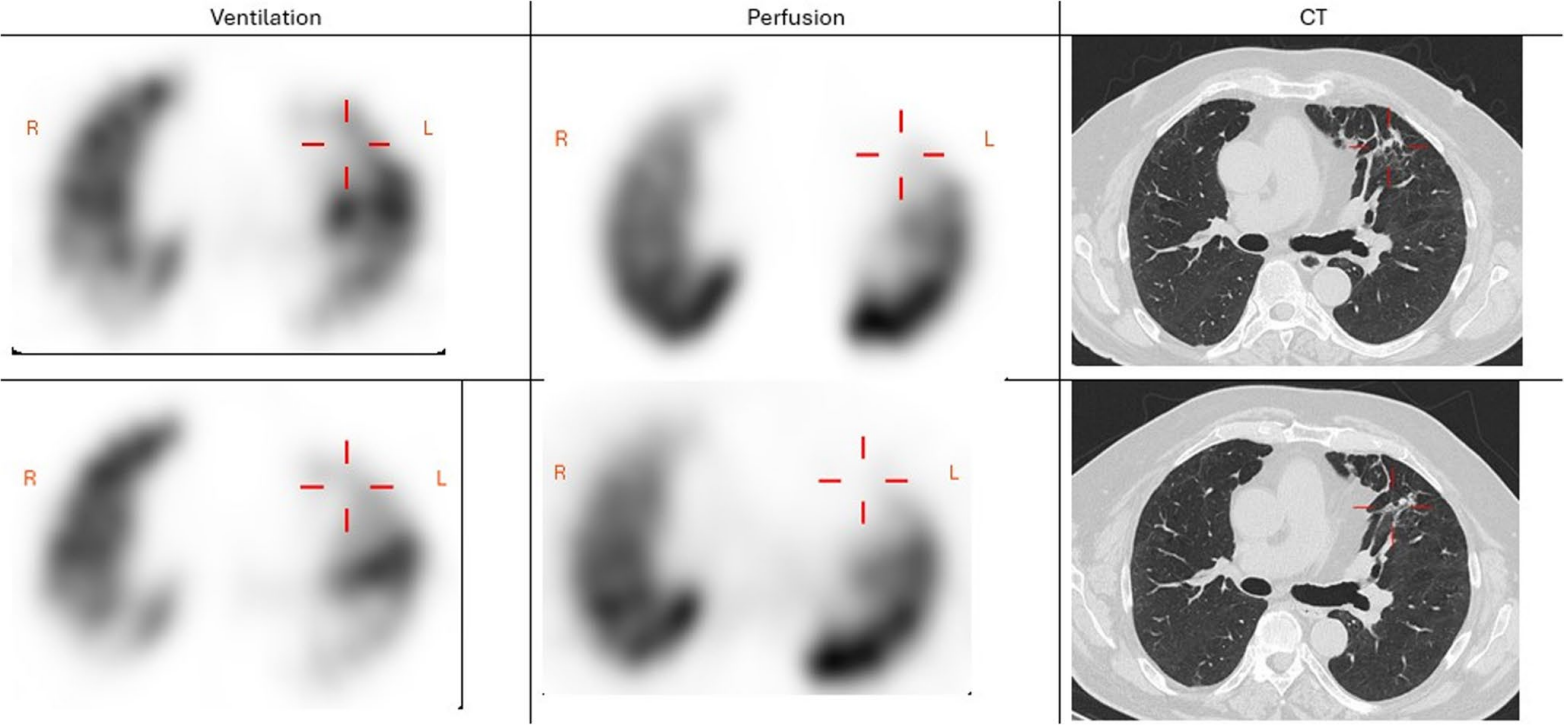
Chest imaging findings at short-term and intermediate follow-up in a 71-year-old male. Ventilation-perfusion single-photon emission computed tomography (V/Q SPECT) with high-resolution computed tomography (HRCT) shows a subsegmental mismatched perfusion defect with slightly reduced ventilation in the left segment 3 (red crosses on axial projections), both at short-term follow-up (6 months after COVID-19; upper row) and at intermediate follow-up (13 months after COVID-19; lower row). At both timepoints, HRCT shows reticulation, fibrosis and emphysema, but these are less pronounced at intermediate follow-up, indicating that the mismatched perfusion defect is caused by fibrosis rather than thromboembolic disease. Also, hypoventilation with inverse mismatched ventilation defects are seen in the posterior parts of both lungs at both short-term and intermediate follow-up. Overall, the chest imaging findings are compatible with a primary ventilatory disturbances due to fibrosis-like changes in the lung. Accordingly, lung function testing showed moderate restriction with a total lung capacity that was 67% of predicted at both time points, while the forced vital capacity improved slightly from 74% to 80% of predicted, and the pulmonary diffusing capacity improved from 59% to 67% of predicted
